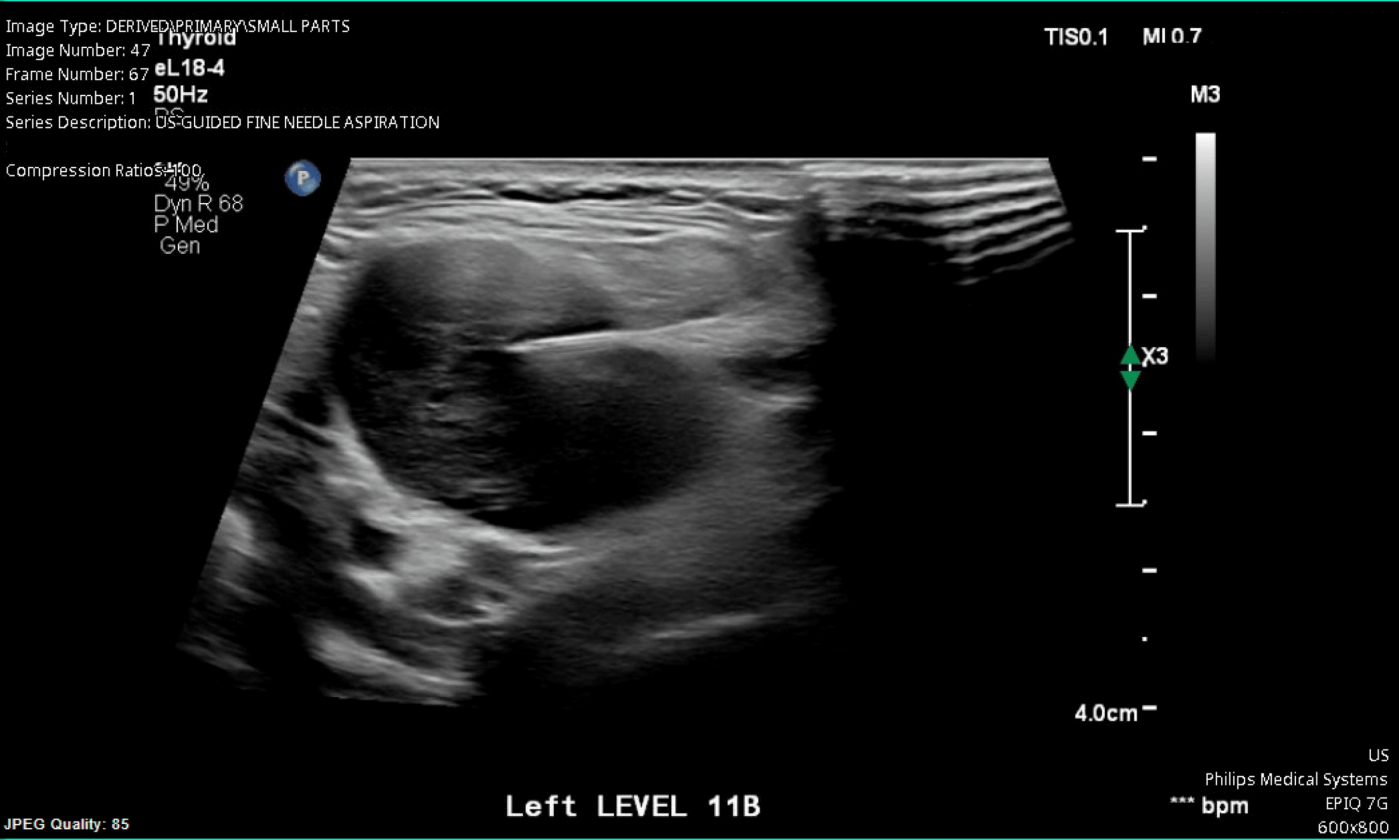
Ultrasound-guided FNA of the neck mass Ultrasound was used to guide the fine needle aspiration of the neck mass. The 18-gauge needle appears as a hyperechoic line, piercing into the center of the neck mass lesion. The needle is inserted into the lesion, and samples of the tissue are obtained percutaneously, and analyzed in a pathology laboratory for malignant or atypical cells. A biopsy is essential to determine if the malignant cells are differentiated or related to the current disease, or, in the unlikely case, if this is an unrelated primary malignancy of the lymph node. FNA: fine needle aspiration
